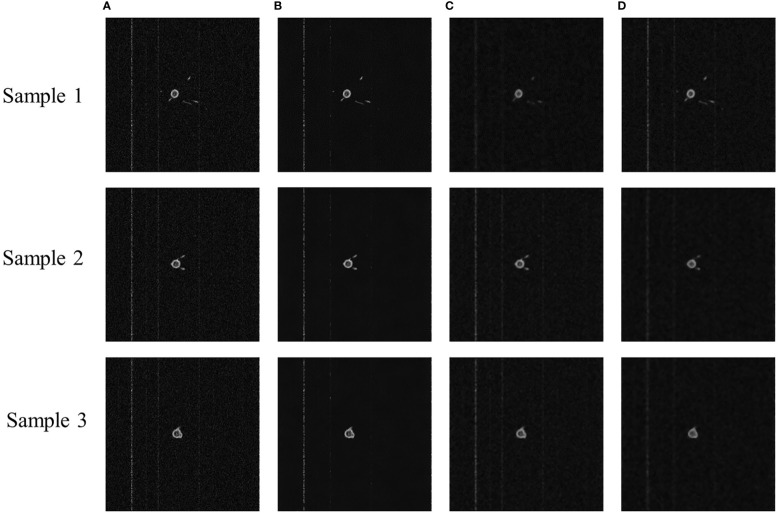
Sample images of denoised MRI images of cotton root system. (A) Original MRI image, (B) NLM denoised image, (C) GLPF denoised image, and (D) MF denoised image.
